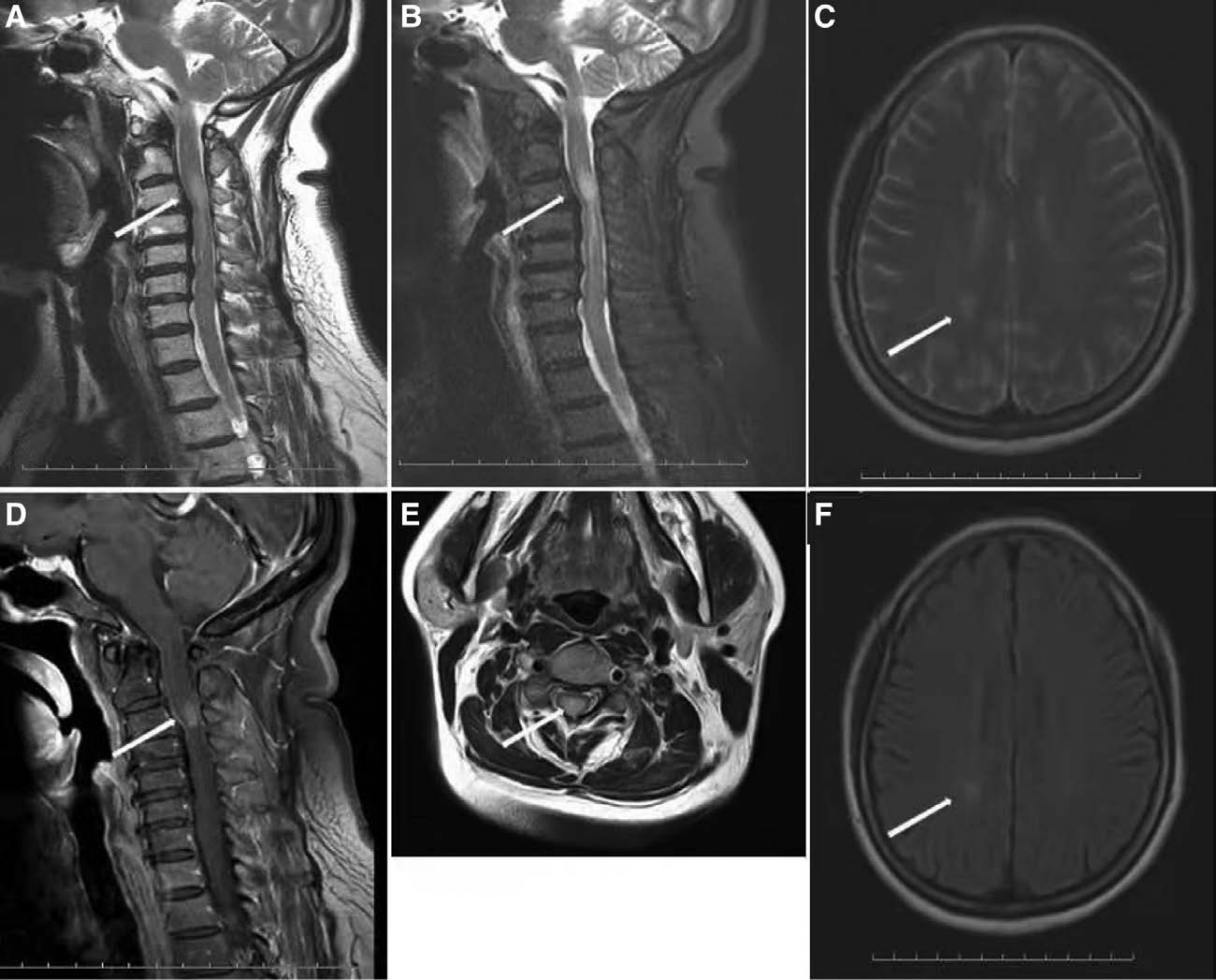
Sagittal magnetic resonance T2-weighted (A) and fat suppression (B) images showing a strip of hyperintensity in the cervical cord (C3–C7) with spinal cord swelling, the edge of the lesion is blurred. Enhanced scan (D) image showing enhancement. Axial T2-weighted (E) image showing small hyperintensities in the cervical cord. Brain axial T2-weighted (C) and Flair (F) images showing 1 nonspecific hyperintense lesion next to the lower corner of lateral ventricles.
